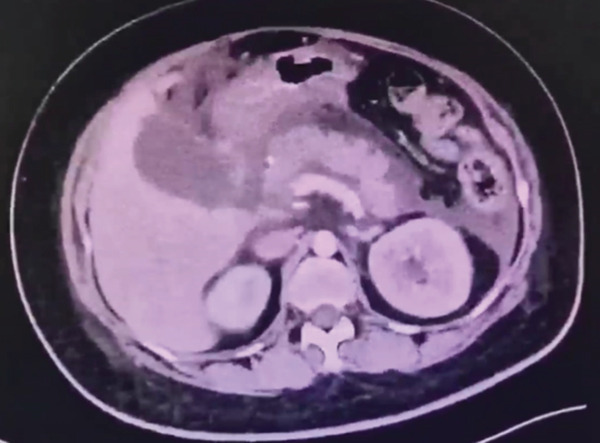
Repeat contrast‐enhanced abdominal CT showing increased peripancreatic inflammatory exudation and the presence of ascites, indicating progression of acute pancreatitis.
